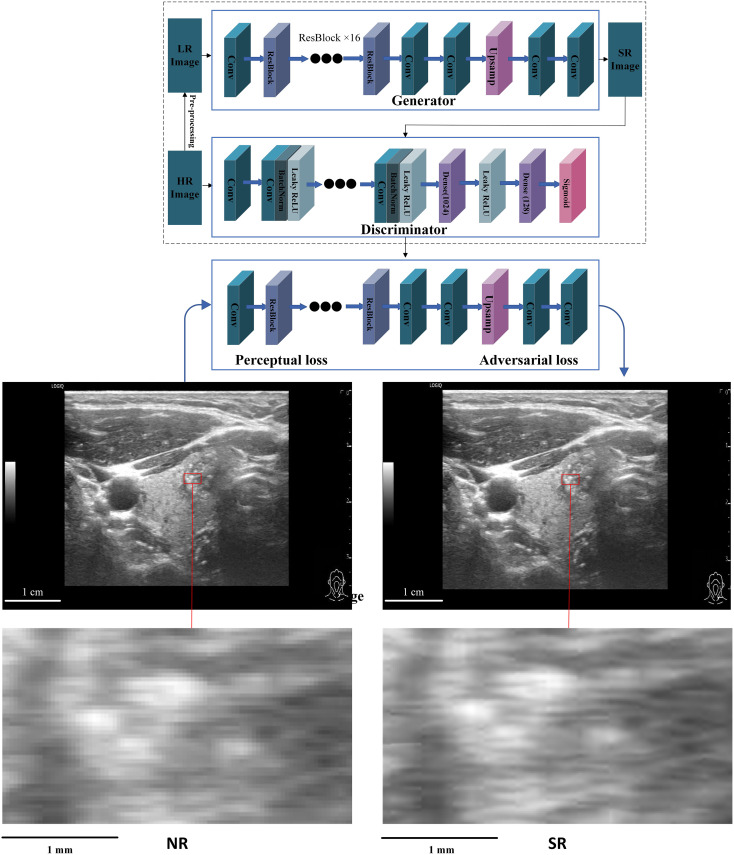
GAN-based super-resolution pipeline and NR–SR image comparison.Top: GAN-based SR pipeline. Middle: NR (left) and SR (right) images of the same nodule. Red boxes indicate regions magnified below for visual comparison (not ROIs). SR denotes GAN-based computational upscaling/detail enhancement of B-mode ultrasound. Scale bars: 1 cm (original), 1 mm (magnified). NR, normal-resolution; SR, super-resolution; GAN, generative adversarial network.
